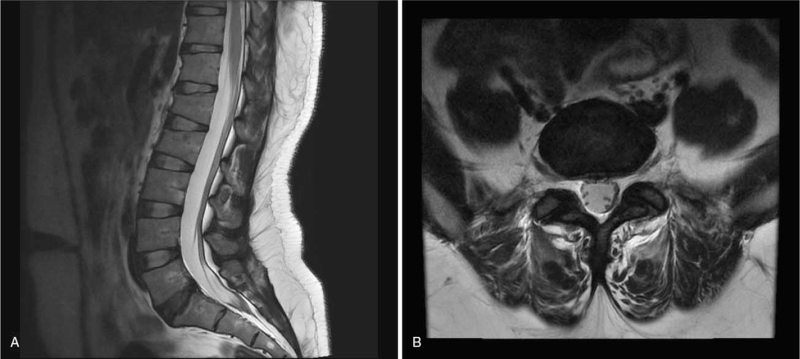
Sagittal and axial T2-weighted lumbar spine magnetic resonance imaging at 2 months after pain onset showing disc bulging and annular fissure without a high-signal intensity zone at L5-S1.
